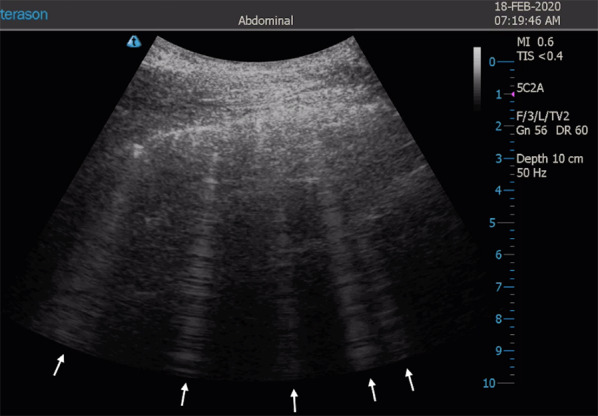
Sonographic appearance of B-lines in oblique view. White arrows indicate multiple B-lines
